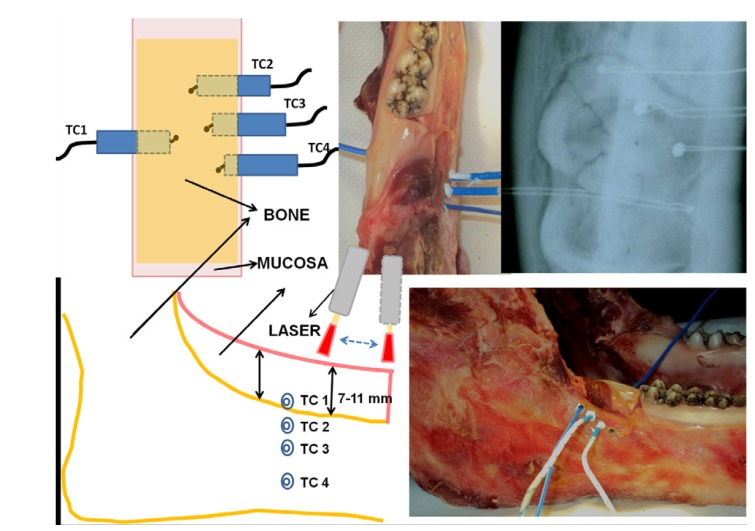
Graphical representation and pictures of placement of 4 thermocouples and X-ray control of their right position. Nd:YAG laser application and Thermal Camera monitoring of surface temperature.
